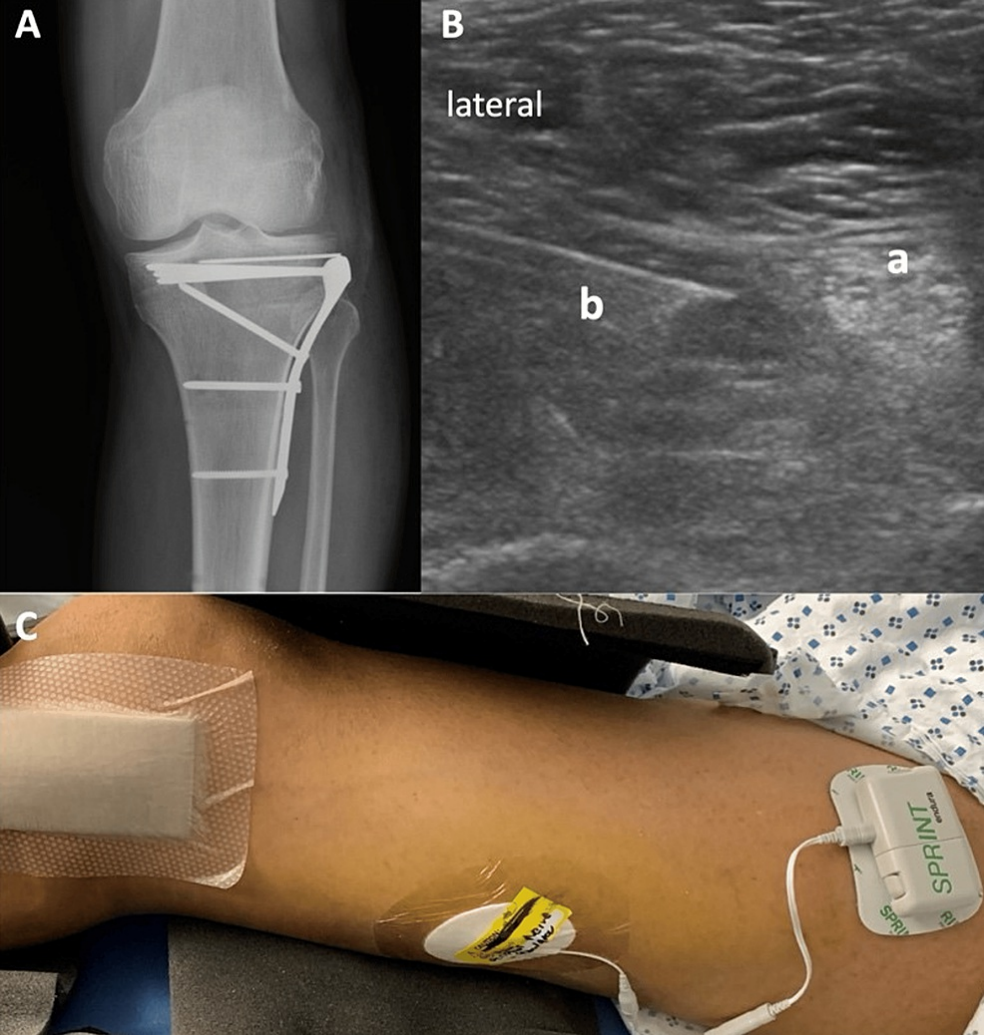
Images of peripheral nerve stimulation (PNS) placement Figure 1: (A) Postoperative X-ray imaging following open reduction and internal fixation of the tibial plateau fracture, (B) Ultrasound-guided needle/electrode placement for PNS placement, and (C) the PNS system a: Sciatic Nerve, b: Needle
